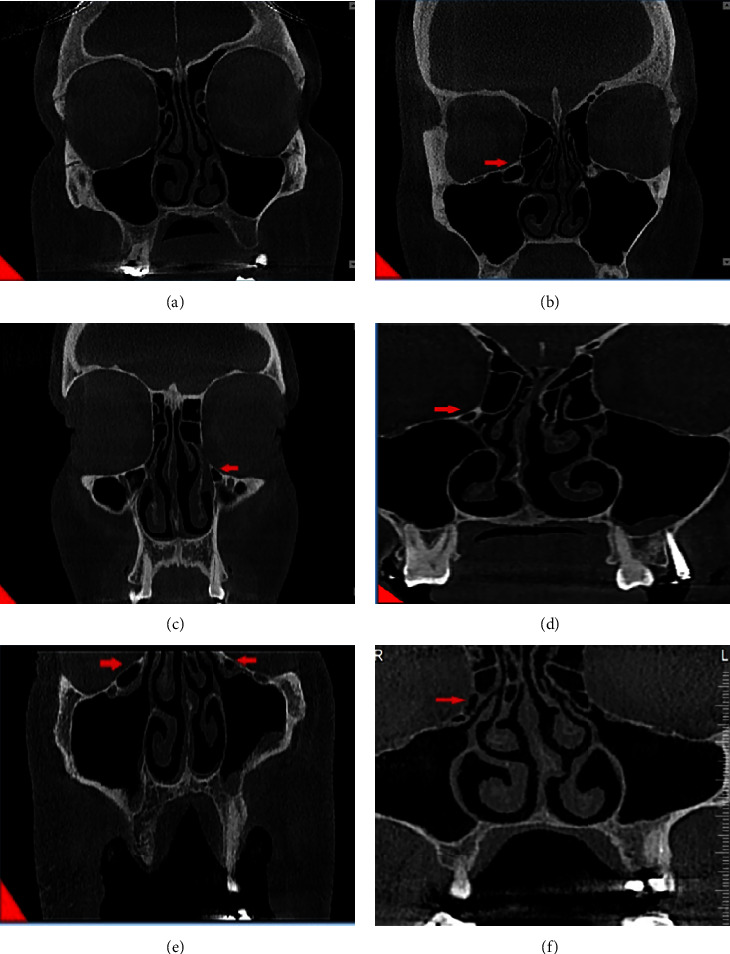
Detection of Haller cell in coronal view CBCT. (a) No Haller cell is detected. (b) Large Haller cell on the right side. (c) Medium sized Haller cell on the left. (d) Small-sized Haller cell on the right. (e) Bilateral presence of Haller cell with two different sizes. (f) Dehiscence of inferomedial border of orbit due to the presence.
